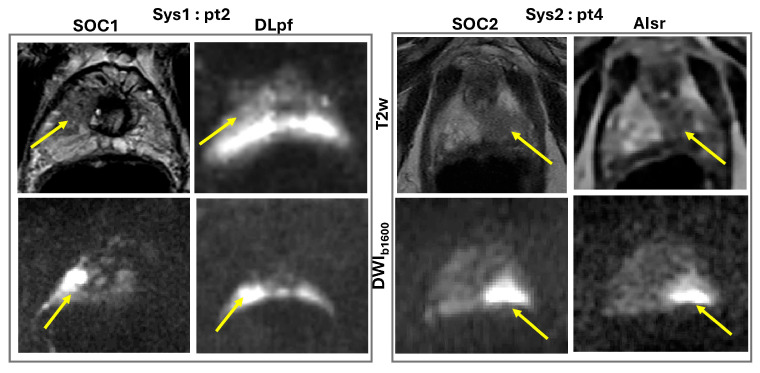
Different sensitivity to susceptibility distortions for standard of care (SOC1/SOC2) versus DL/AI accelerated bi-parametric (bp)MRI on two 3T systems (Sys1 and Sys2) for two patients (pt) with peripheral zone PIRADS 5 lesions (arrows) in the right apex (pt2) and left apex (pt4).
